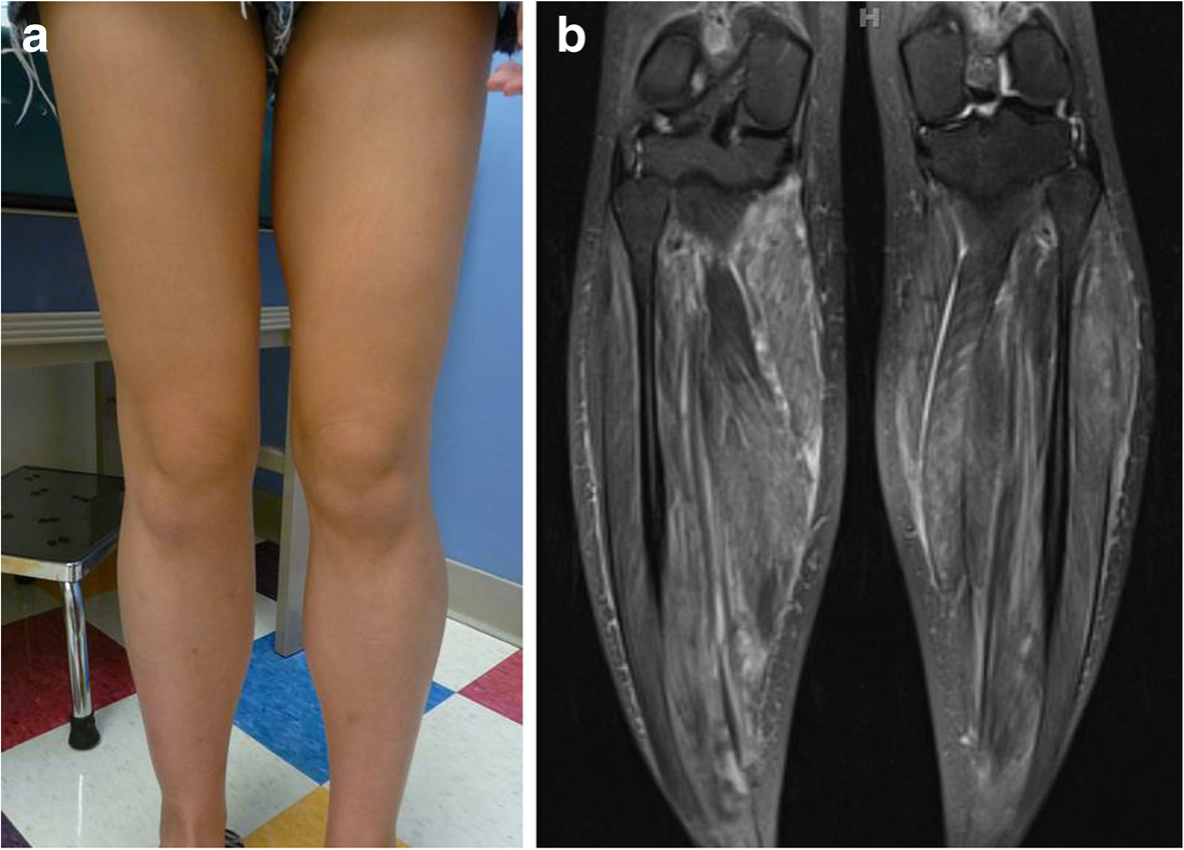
a Photograph of lower extremity hypertrophy. b Comparison to coronal T2 MRI showing symmetric hypertrophy of lower extremity musculature
